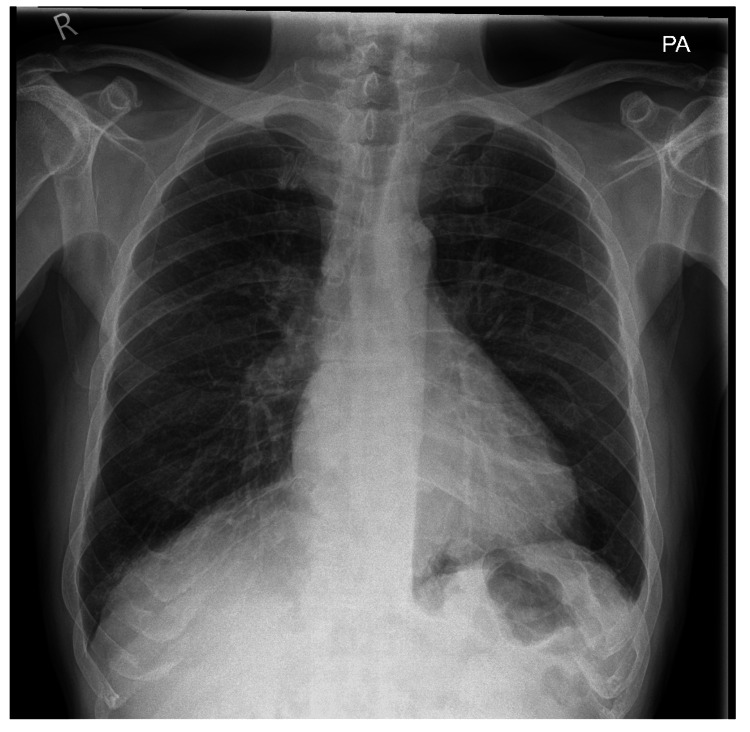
Follow-up outpatient department chest X-ray done at four months after discharge.
